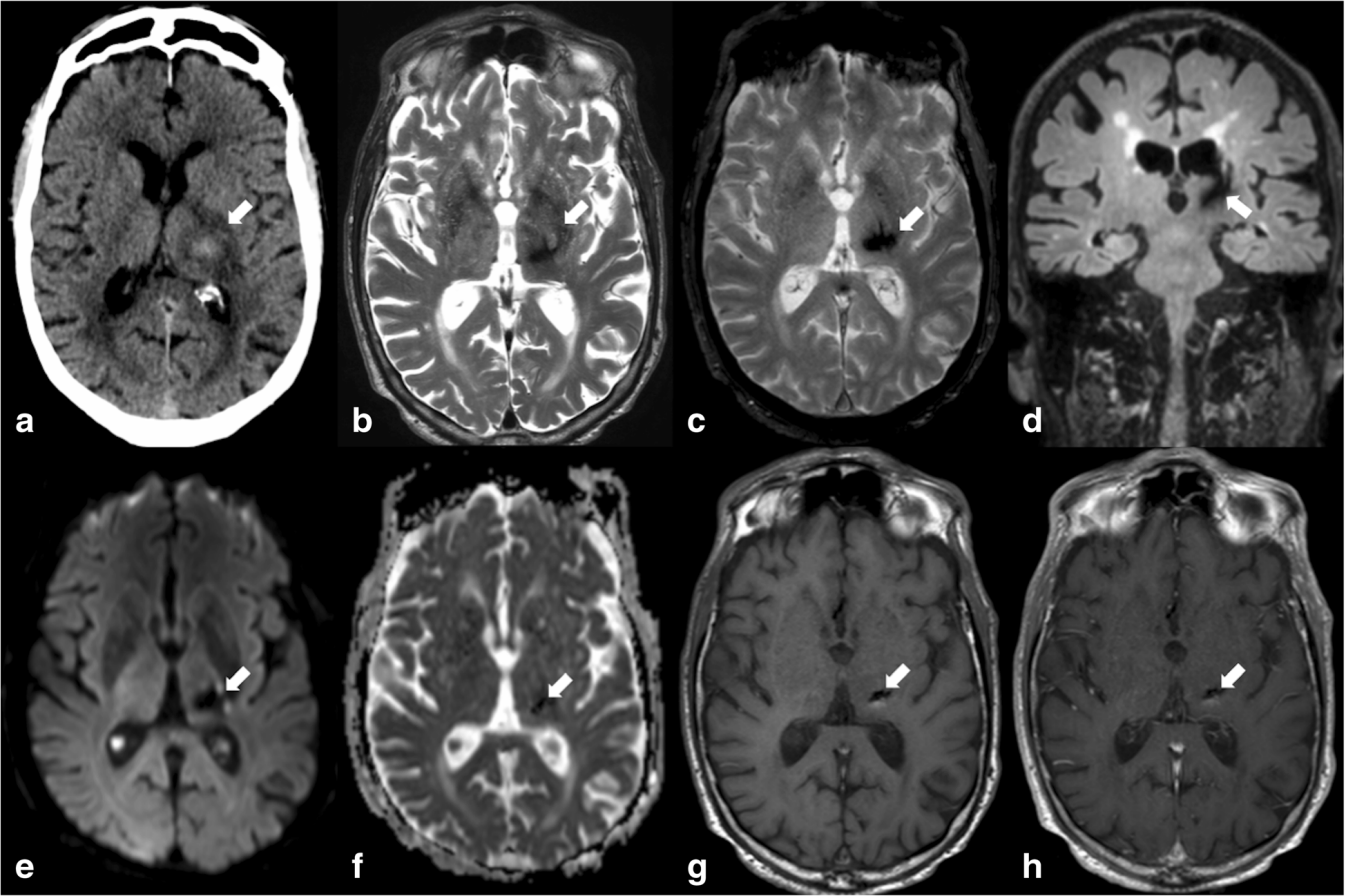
There is a hyperdense lesion on non-contrast surrounded by oedema (arrow in a). Follow-up MRI shows resolution of the oedema and low signal intensity of the lesion on all sequences including T2 (b), T2* (c), FLAIR (d), DWI b1000 (e), ADC map (f), non-contrast T1 (g), and T1 post contrast (h) reflecting the expected progression of a subacute into chronic haematoma in a patient with hypertension
